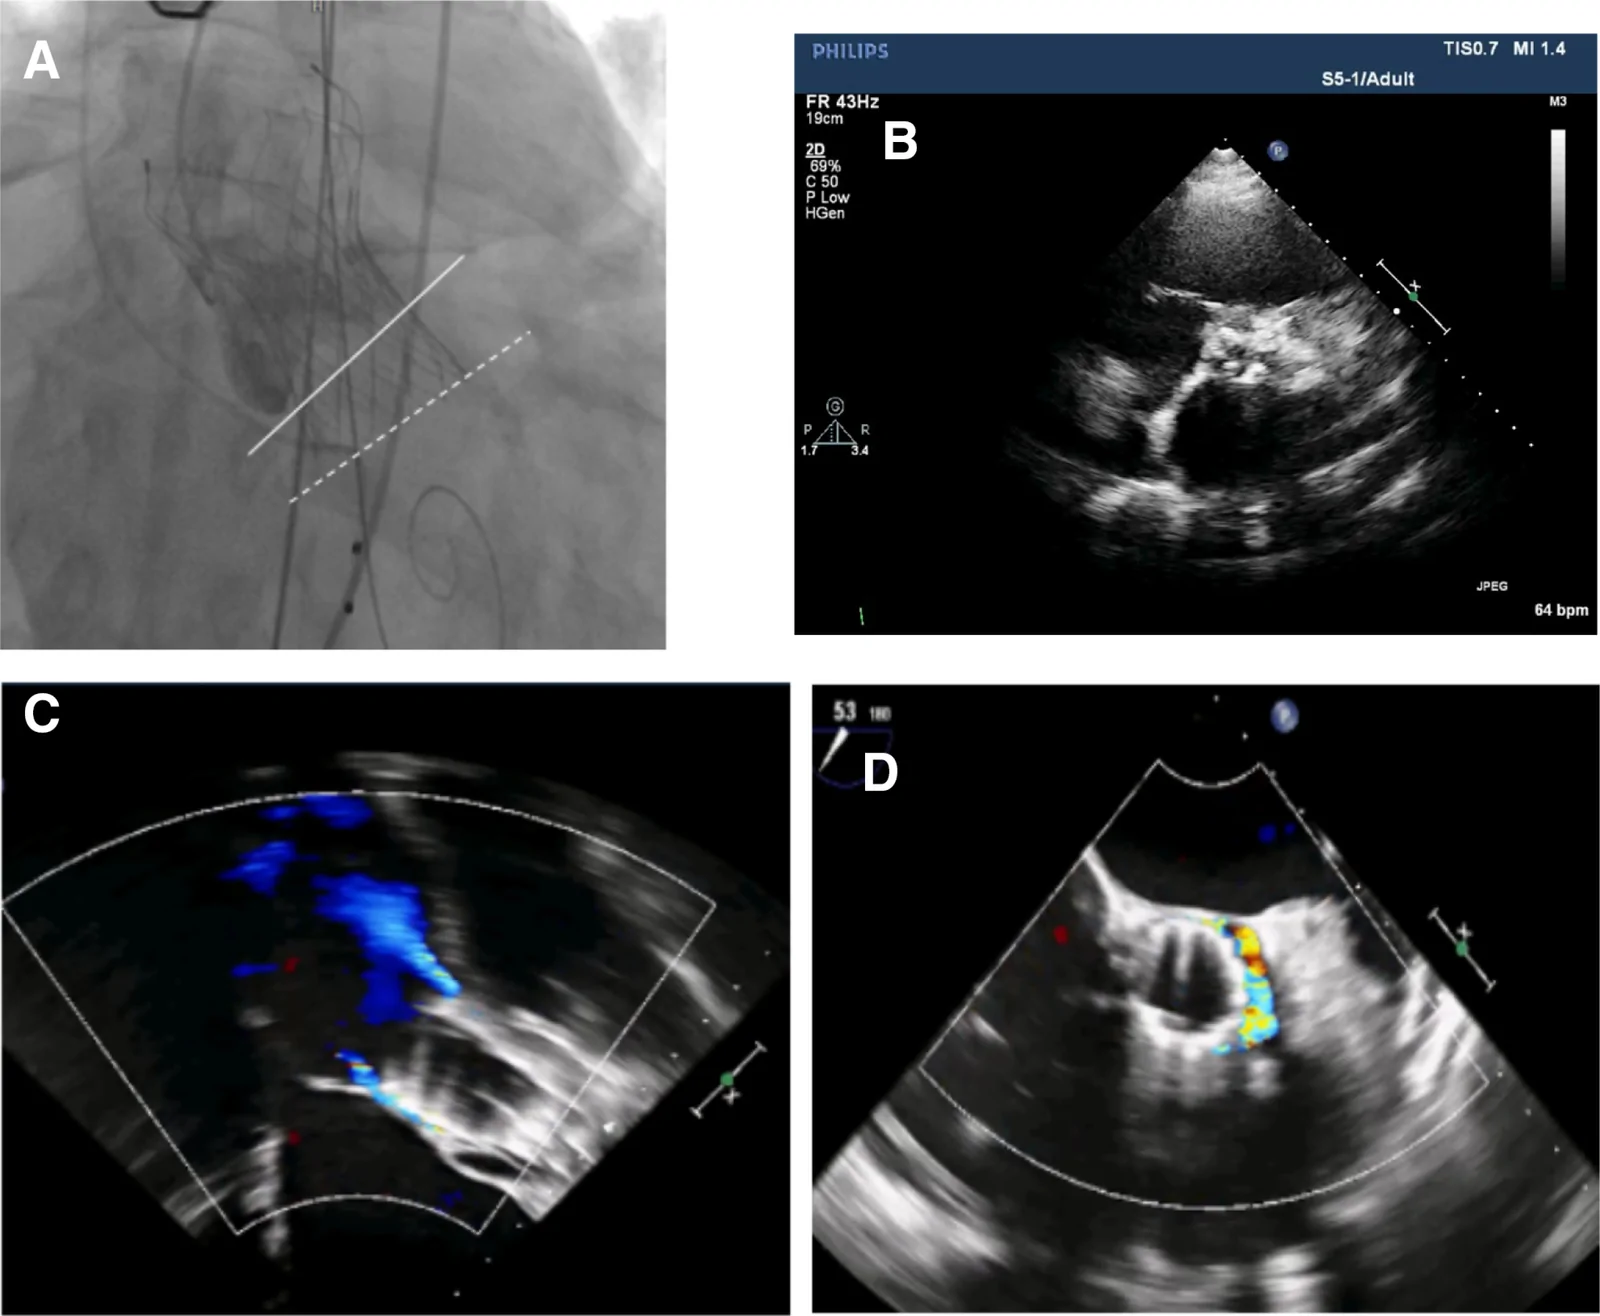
Digital subtraction angiography (DSA), transesophageal echocardiography (TEE), and transthoracic echocardiography (TTE) evaluation during the 1st transcatheter aortic valve replacement (TAVR) operation. The solid white line shows the plane of the valve ring, and the white dotted line shows the plane of the bottom of the valve prosthesis (A). Preoperative TTE evaluation showing severe aortic stenosis and regurgitation (B). The postoperative TEE evaluation results showed mild and moderate perivalvular leakage (PVL) (C) in the long-axis plane and 2 mm reflux beam (D) in the 1 and 5 o’clock directions in the short-axis plane.
